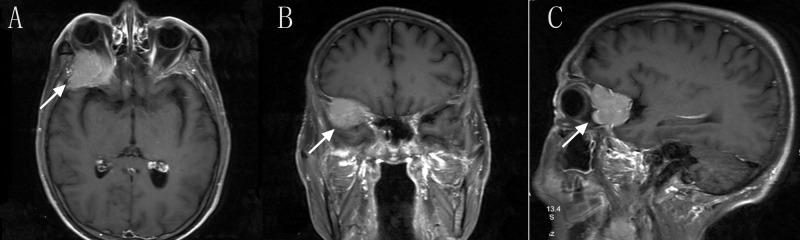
Initial T1 Contrast-enhanced magnetic resonance imaging Axial (A),Coronal (B) and Sagittal (C) T1 Contrast-enhanced MRI imaging revealed an cranio-orbital tumor(arrow).
